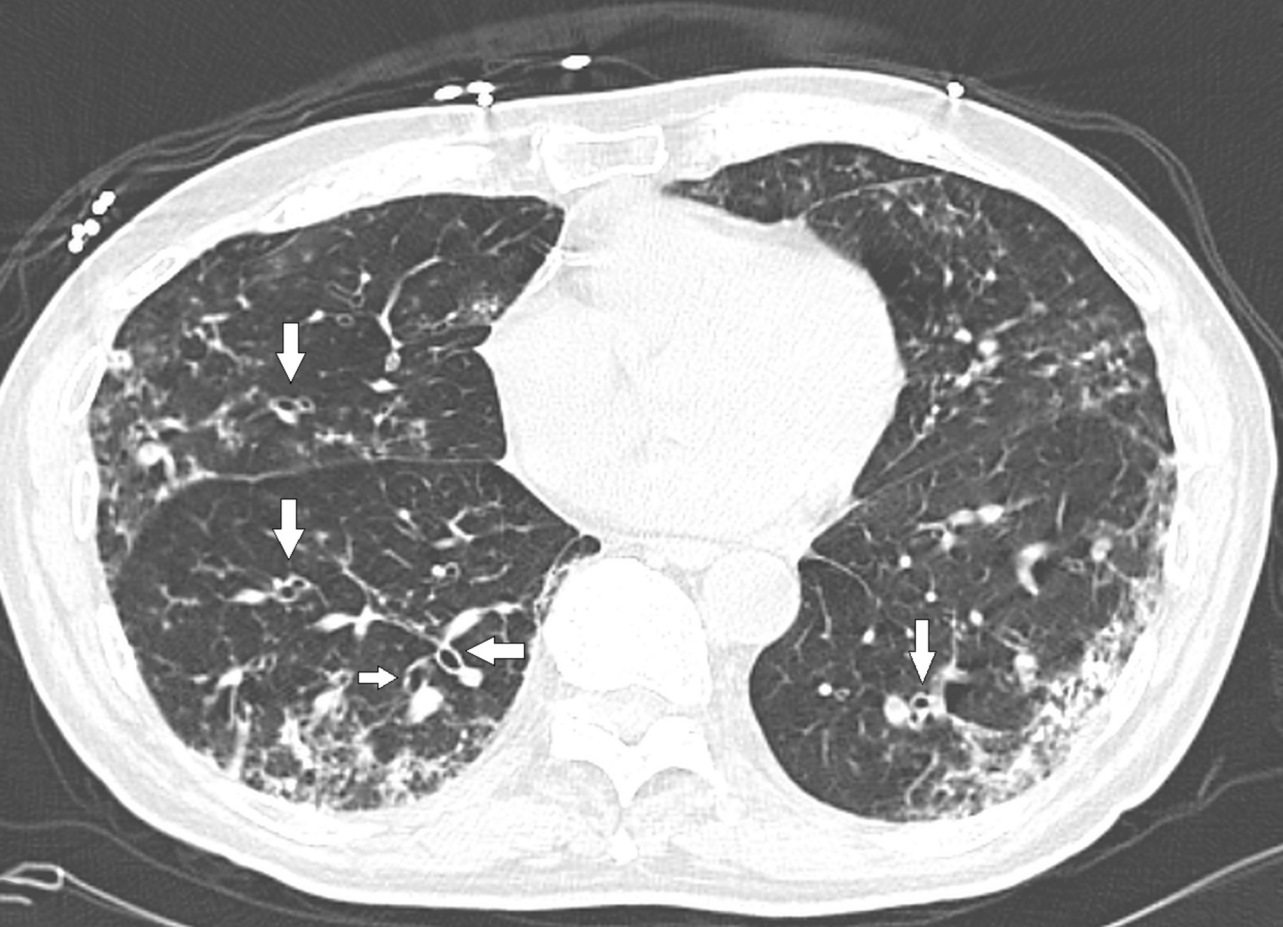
Computed tomography scan showing bronchiectasis
